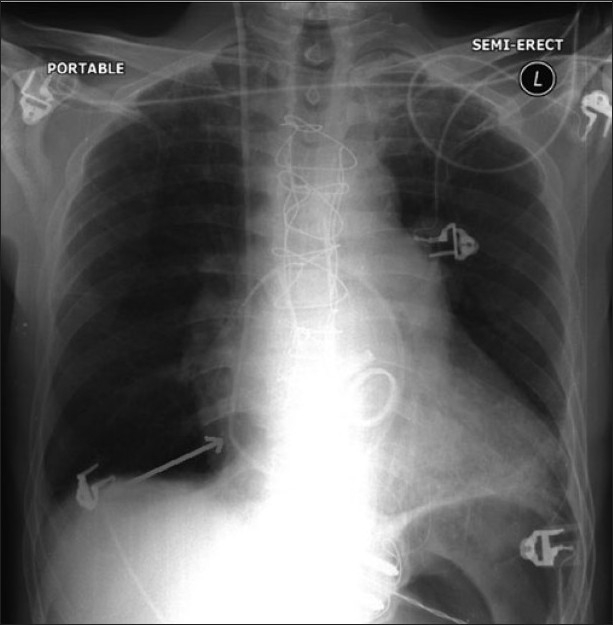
Chest radiograph on the first postoperative day. Arrow shows angulation of the PAC
